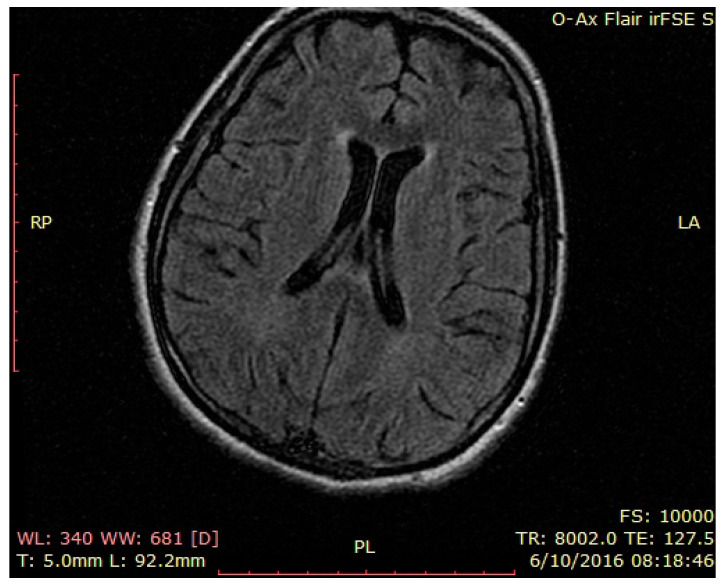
Second brain MRI, FLAIR images, axial parietal image.
